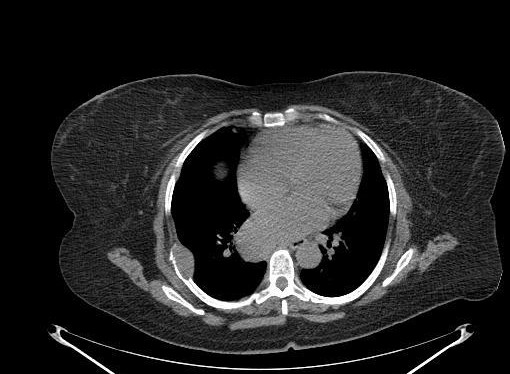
CT scan of chest showing mediastinal mass and pathological fracture of the right 8^th ^rib.
